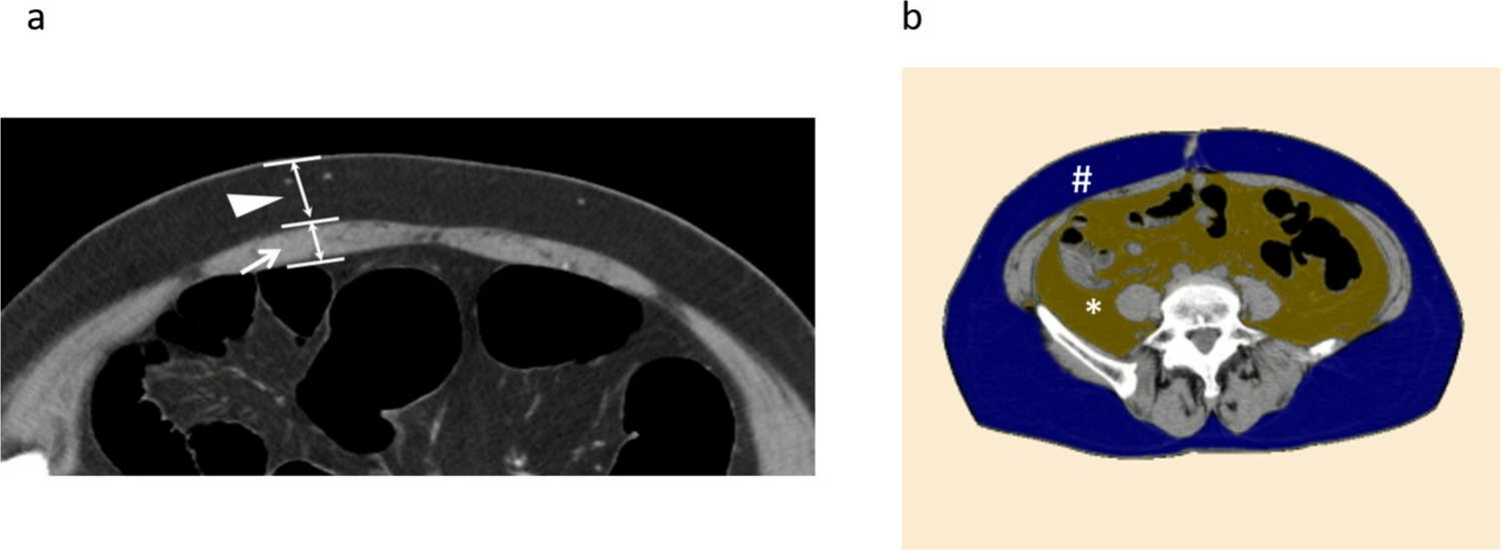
Measurement of TAM, TSF, AVF, and ASF. a TAM and TSF were measured as vertical distances at the planned ileostomy site on preoperative CT. b ASF and AVF were calculated at the level of the umbilicus using the SYNAPSE VINCENT 3D image analysis system. Arrow: TAM distance; arrowhead: TSF distance; #, blue area: ASF; *, yellow area: AVF. *TAM* thickness of the rectus abdominis muscle, *TSF* thickness of the subcutaneous fat, *AVF* area of the visceral fat, *ASF* area of the subcutaneous fat
